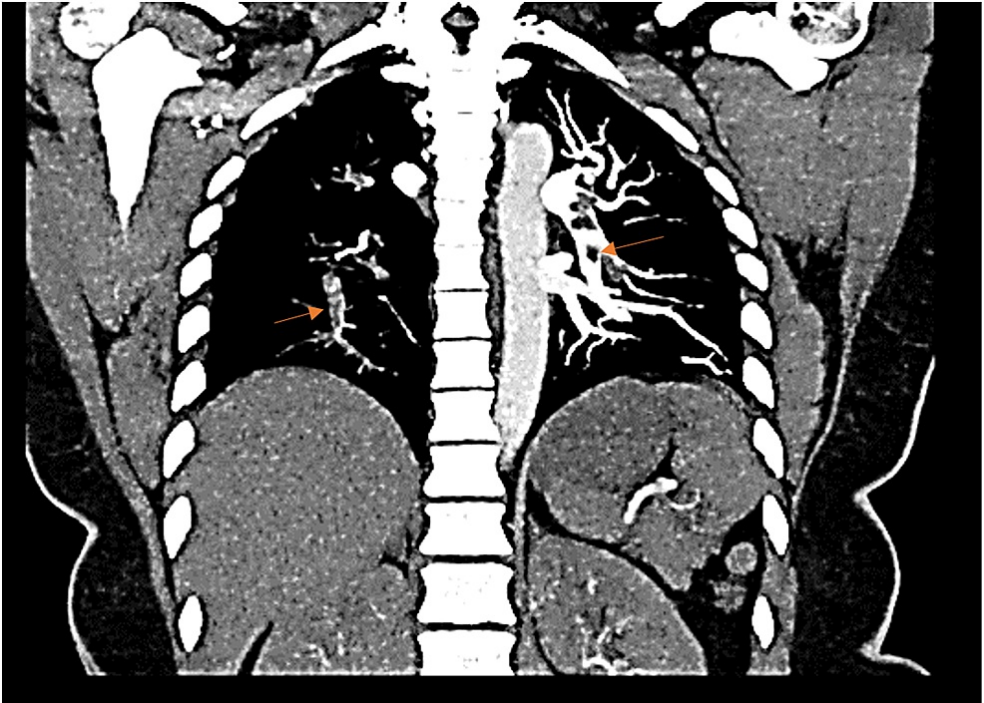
Computed tomographic angiography of the chest (coronal view). Computed tomographic angiography of the chest showing multiple emboli (orange arrows) in the upper and lower lobes bilaterally.
